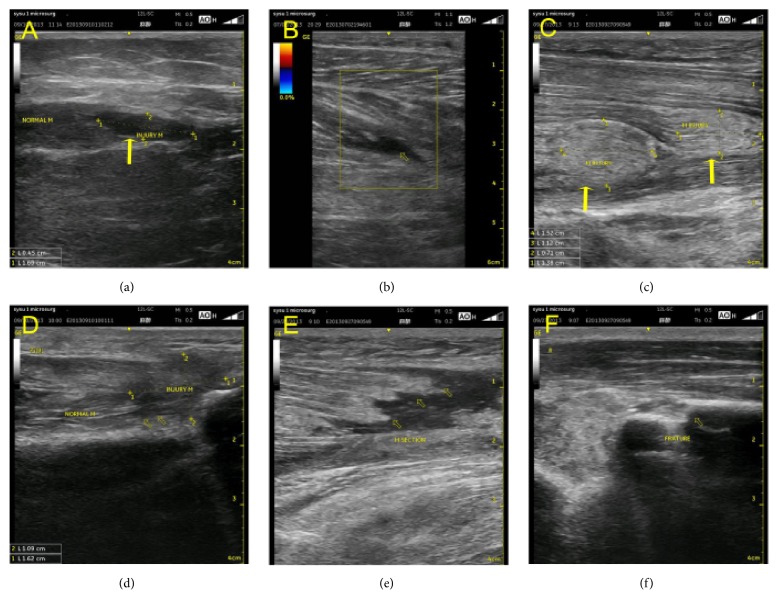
Grade I muscle rupture, but focal fibre rupture with less than 5% of the muscle involved; ultrasonography shows a hypoechoic mass within the muscle fibres (yellow arrow) (a, b). Grade II partial muscle rupture; the muscle belly forms a real mass, with focal fibre rupture with more than 5% but less than 30% of the muscles involved. Ultrasonography shows a hypoechoic mass within the muscle fibres (yellow arrow) (c, d). Grade III lesion represents focal fibre rupture with more than 30% of muscle rupture with retraction, and it also shows the bell-clapper sign, with torn muscle fragments floating in the serohematic fluid (yellow arrow) (e). Ultrasonography shows a limb fracture, fracture of the radius (yellow arrow point) (f).
